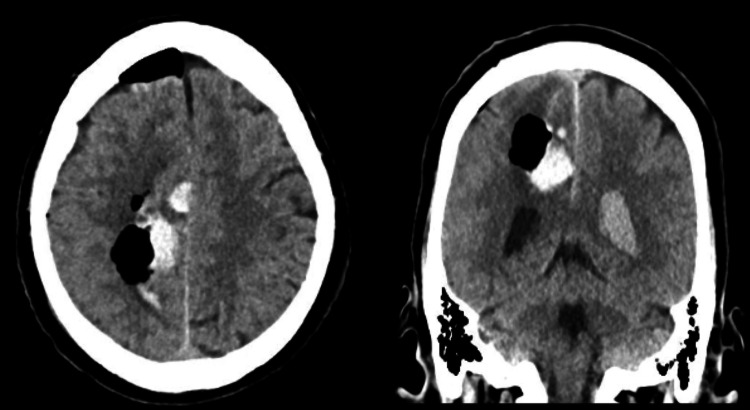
Post-operative CT scan for patient 2 Post-operative CT scan demonstrating excellent evacuation of the right frontal sICH sICH: Spontaneous intracerebral hemorrhage
